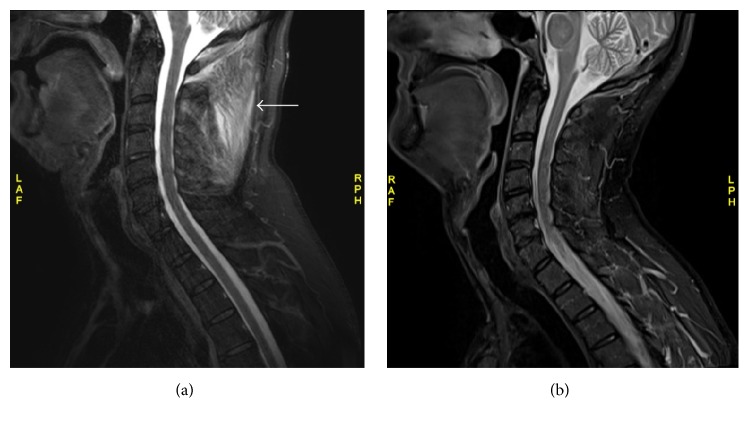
(a) T2-weighted sagittal MRI of neck pretreatment, showing hyperintensity in the posterior paraspinal muscles, consistent with myositis (arrow). (b) T2-weighted sagittal MRI of neck following six weeks of antibiotic treatment, showing complete resolution of myositis.
